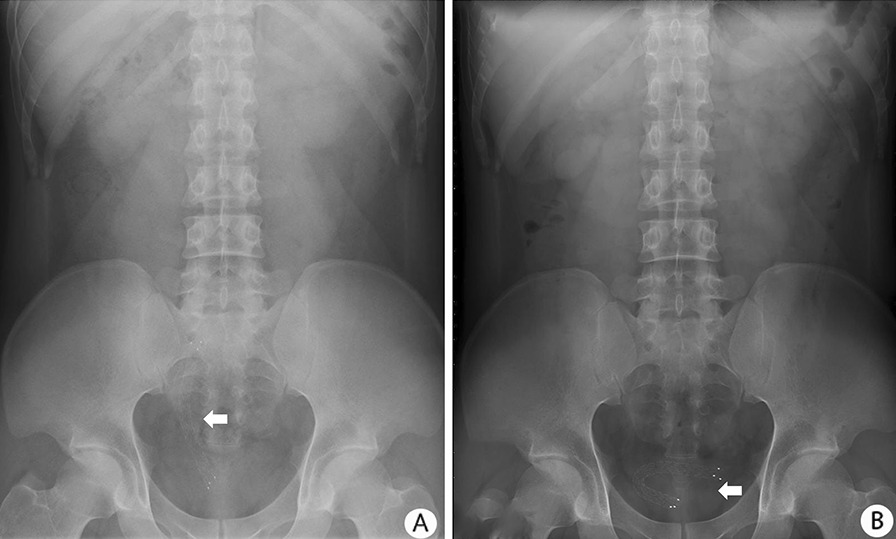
KUB radiography of stent displacement in patient with lower ureteral stricture 1 day and 6 months after insertion: A KUB radiography showing the normal position of stent 1 day after insertion; B KUB radiography demonstrating the stent migration into the bladder 6 months after placement
